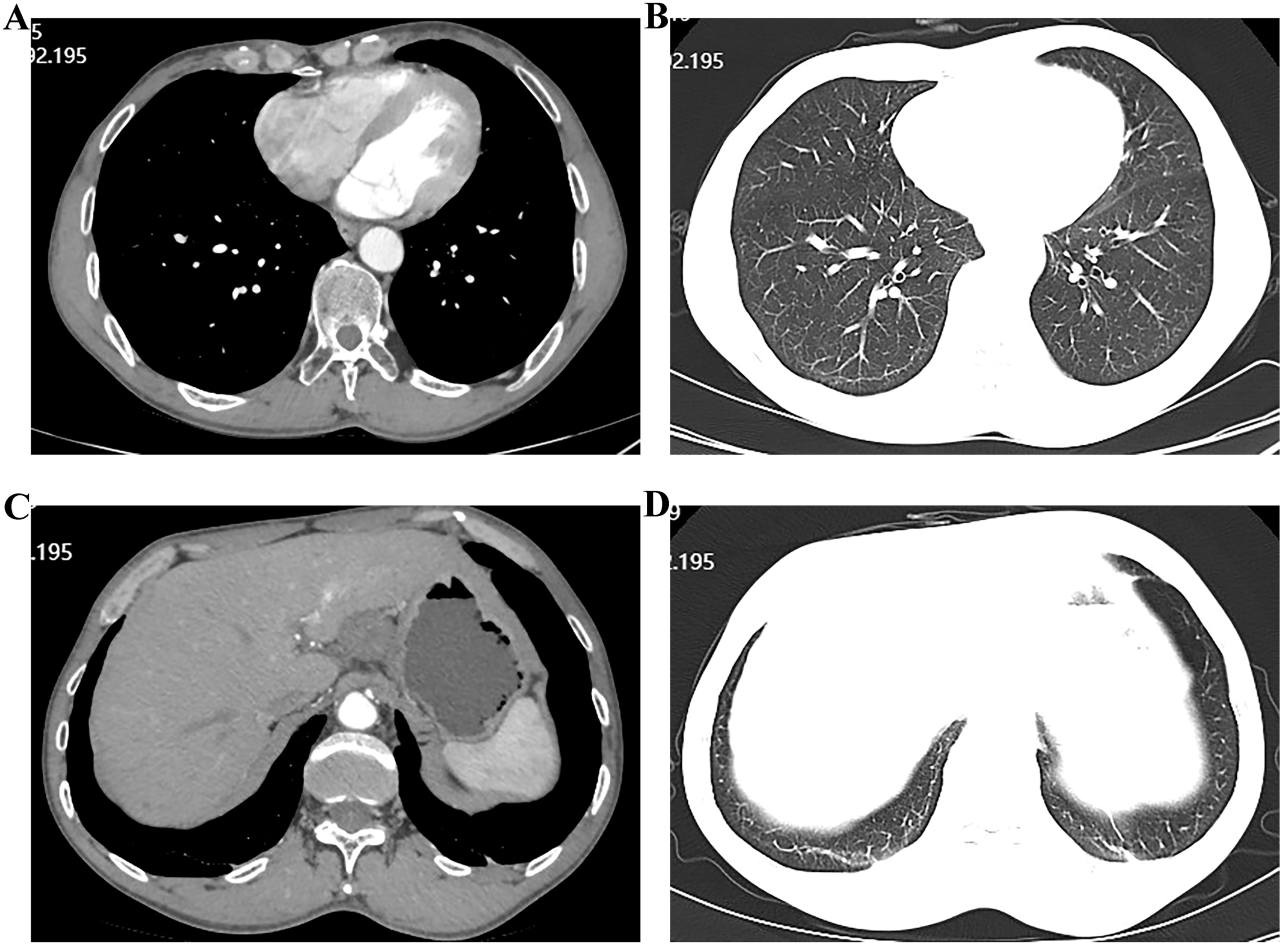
Computed tomographic (CT) images showing esophageal and hepatic gastrointestinal space-occupying lesions. (A, B) esophageal lesion; (C, D) Hepatic gastrointestinal space-occupying lesion.
